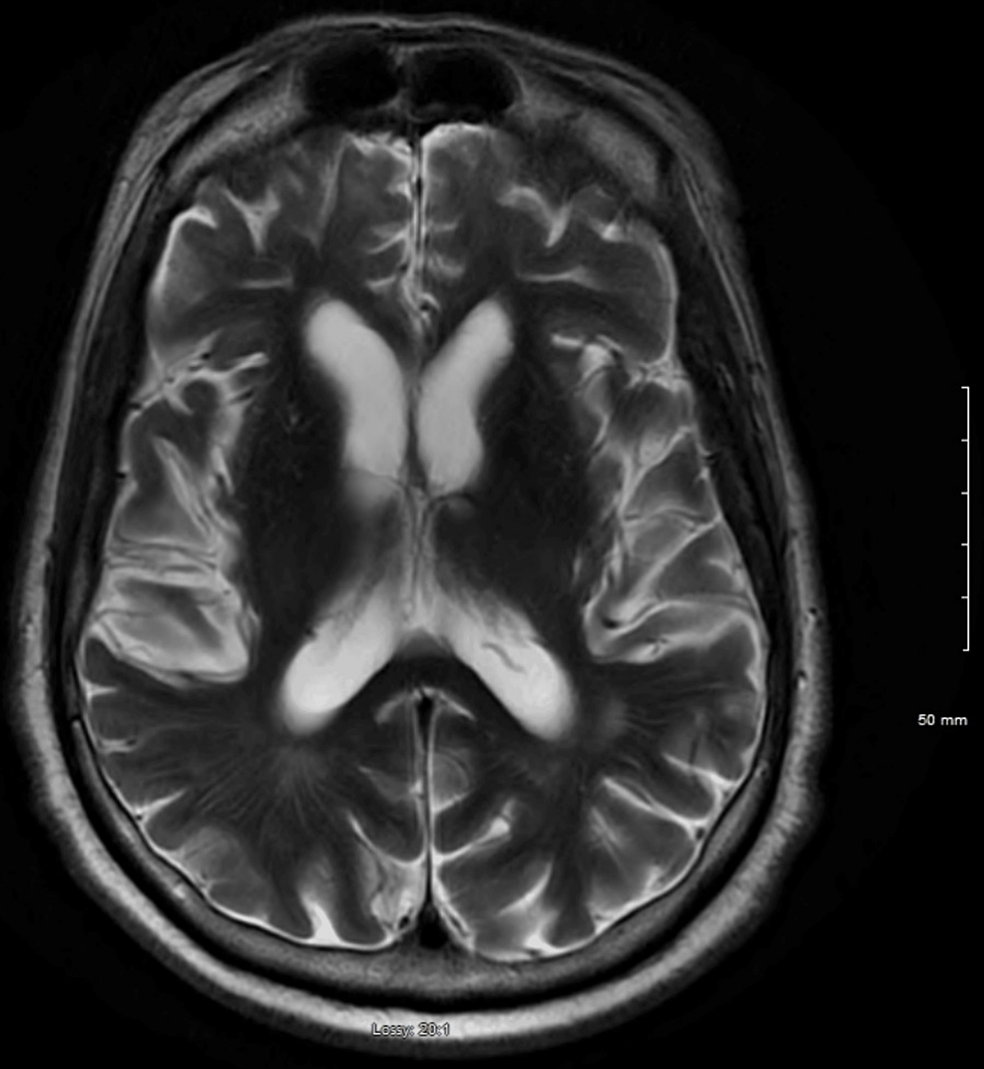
T2 weighted MRI brain with hyperintensity in temporal lobes
